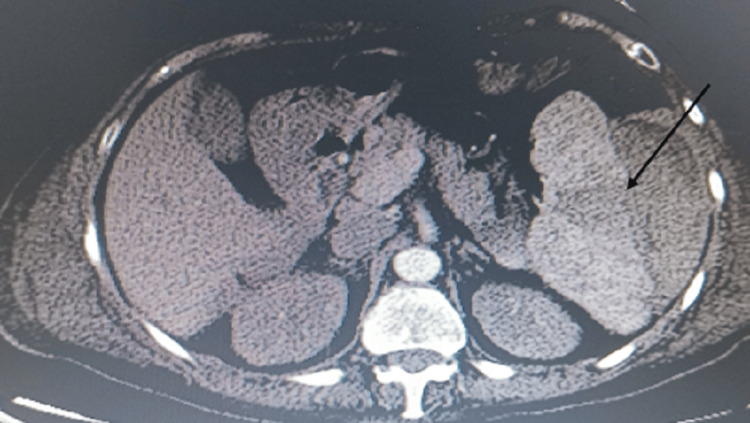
CT scan of the abdomen and pelvis The image shows an effusion of the part of the spleen (black arrow), consistent with splenic rupture CT: computed tomography
